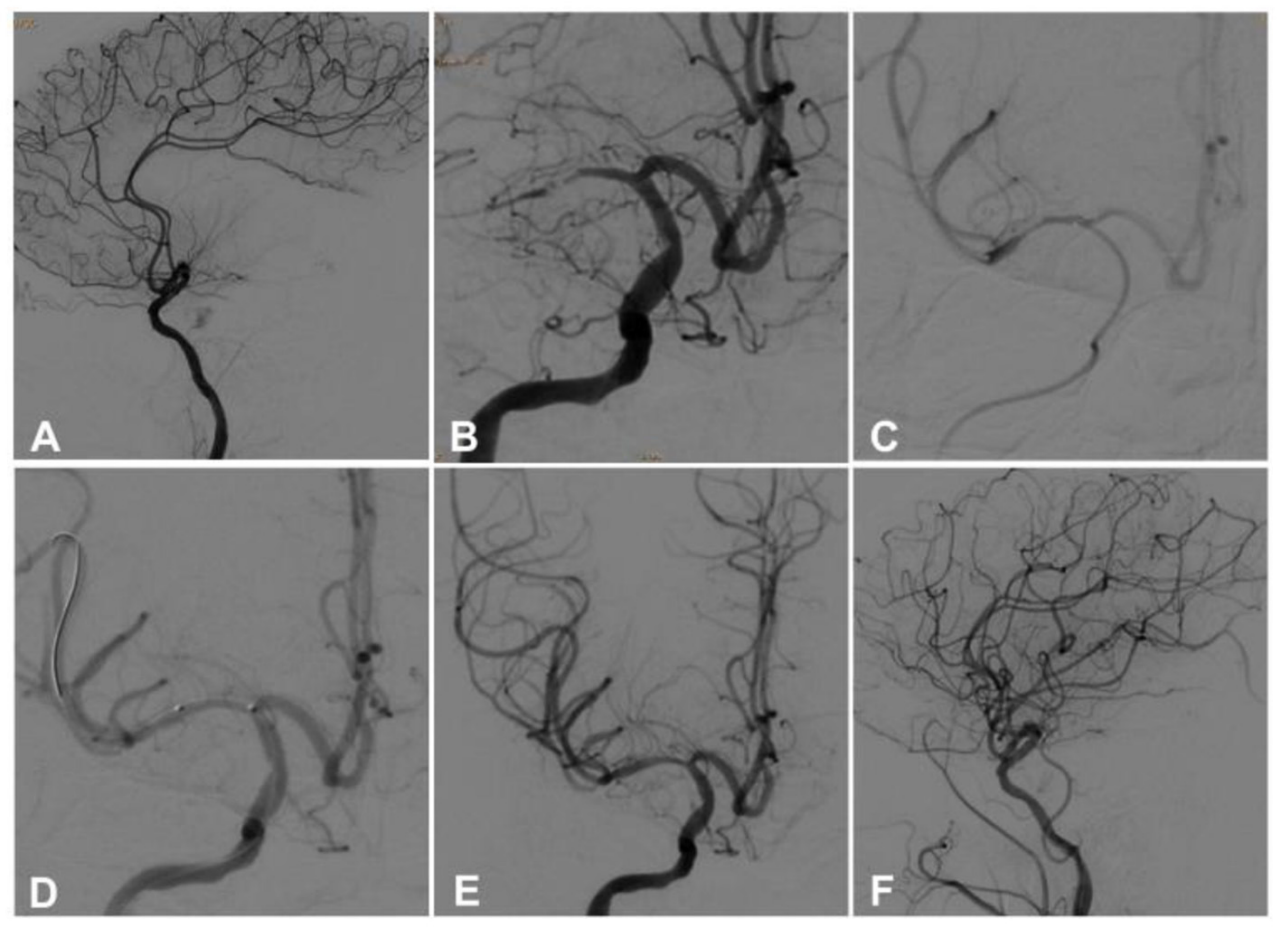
A 36-year-old man had sudden onset of left limb weakness 17 h ago, and the symptom was relieved after intravenous thrombolysis. However, the symptom was aggravated 4 h later, and he was transferred to our hospital with the NIHSS score of 11. Magnetic resonance imaging demonstrated acute infarction in the right basal ganglia. (A,B) Before aspiration, cerebral angiography revealed occlusion of the right middle cerebral artery. (C) After the thrombus was removed by the aspiration catheter connected to the negative pressure pump, the blood flow forward was resumed. (D) During the procedure, a 2 mm × 12 mm balloon was used to expand the stenotic segment. (E,F) An Enterprise stent (4.5 mm × 22 mm) was deployed at the stenotic location, and the blood flow was restored to the modified Thrombolysis in Cerebral Infarction (mTICI) grade 3.
